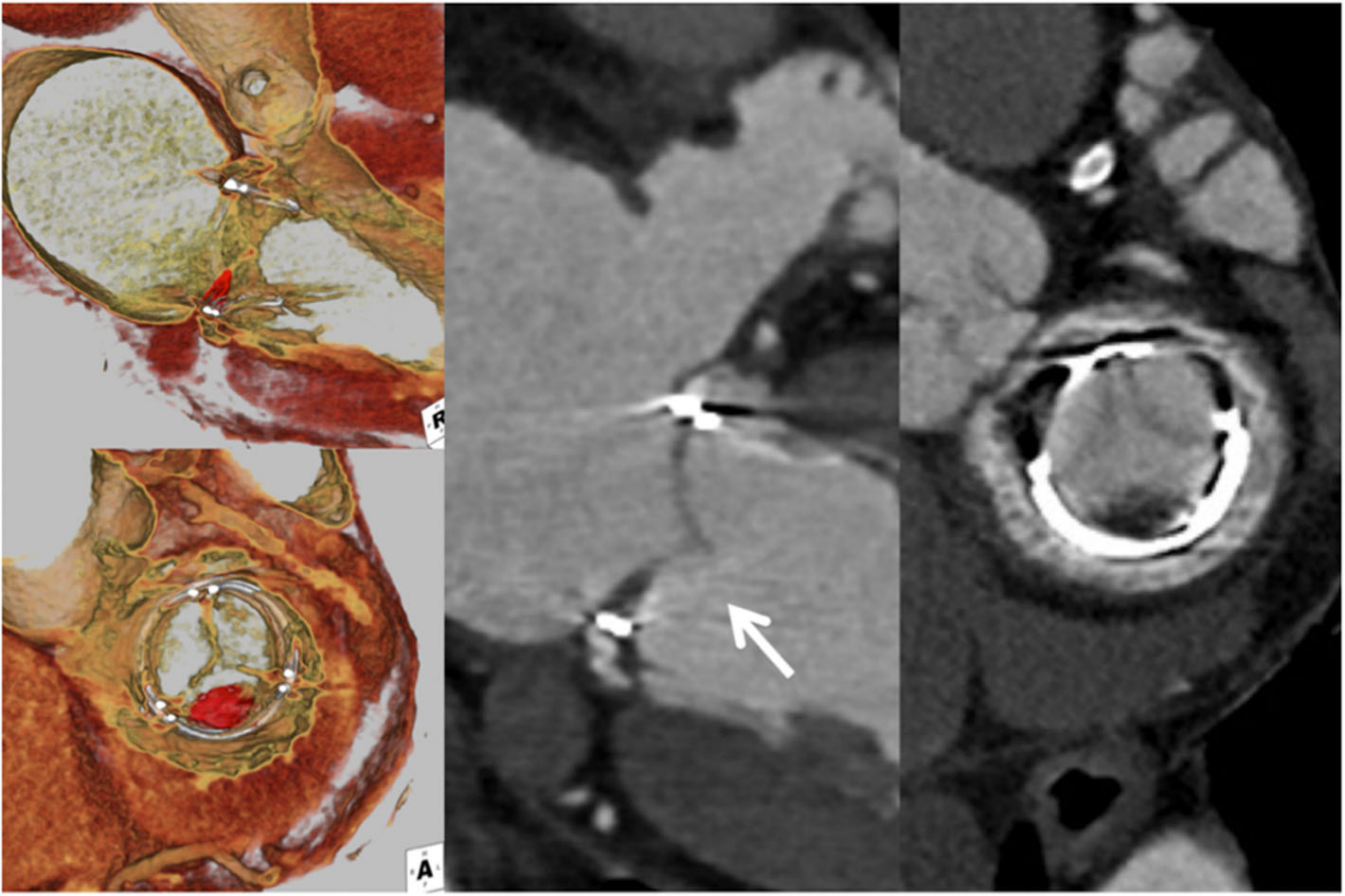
65-year-old male. 3.6 years out of mitral valve replacement. Long-axis and short-axis view. CT image demonstrated a HALT in one leaflet on the periphery near the sewing ring. All panels were obtained on the same day
